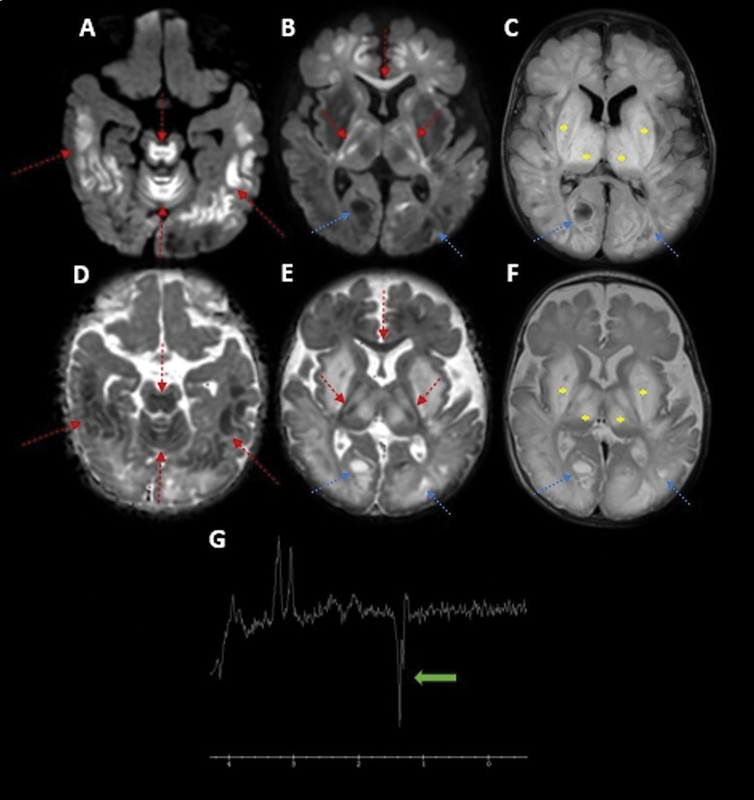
Brain magnetic resonance imaging (MRI) scan at two months of age. Axial diffusion (
